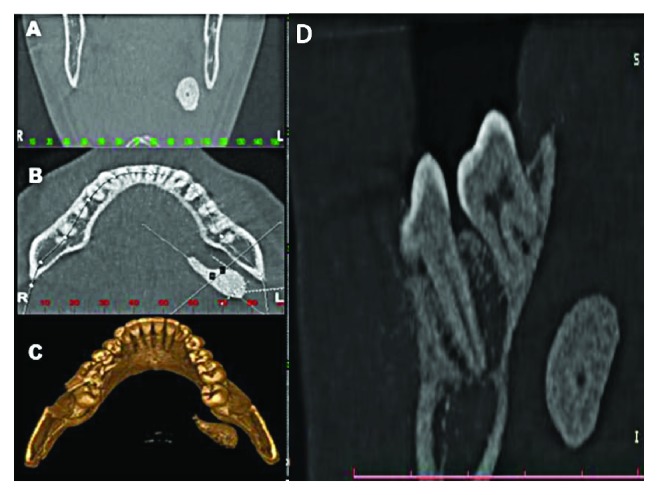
Cone beam computed tomography showing the dimensions of sialoliths. (a) Coronal view. (b) Axial view. (c) 3D reconstruction. (d) Sagittal view.
